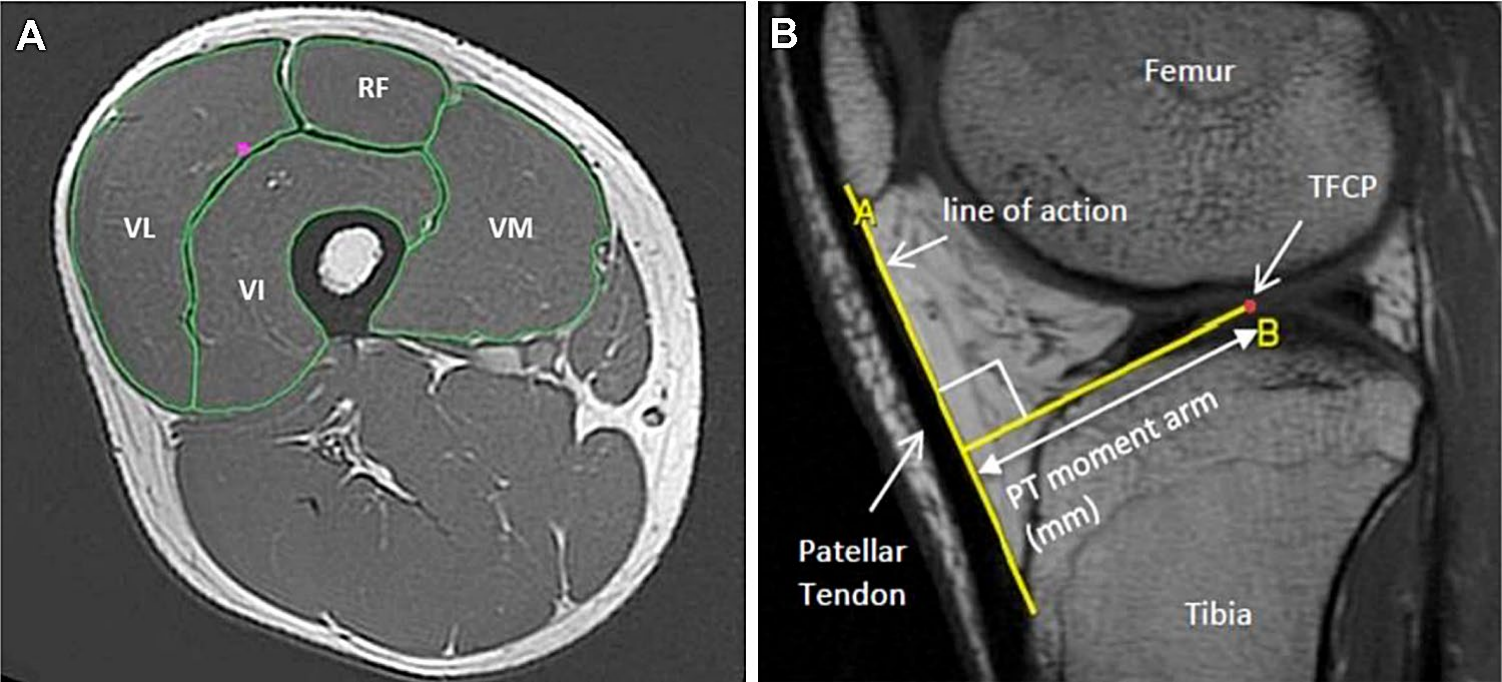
Representative a Axial MR image of the thigh and b Sagittal MR image of the knee joint. Patellar tendon moment arm was defined as the perpendicular distance between the tendon line of action and the tibio-femoral contact point (TFCP)
